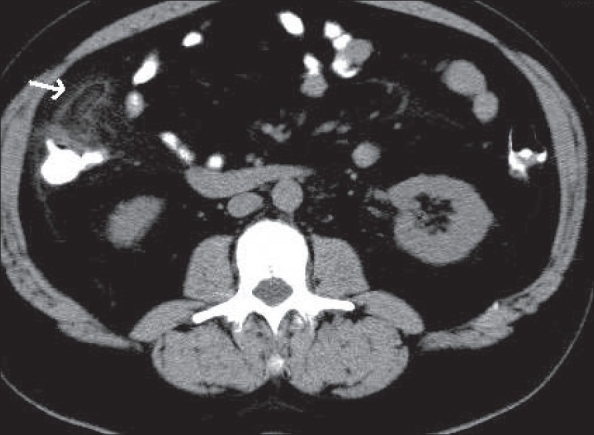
Epiploic appendagitis. Contrast-enhanced CT scan shows a fat density lesion (arrow) with a hyperdense rim and fat stranding adjacent to the ascending colon
